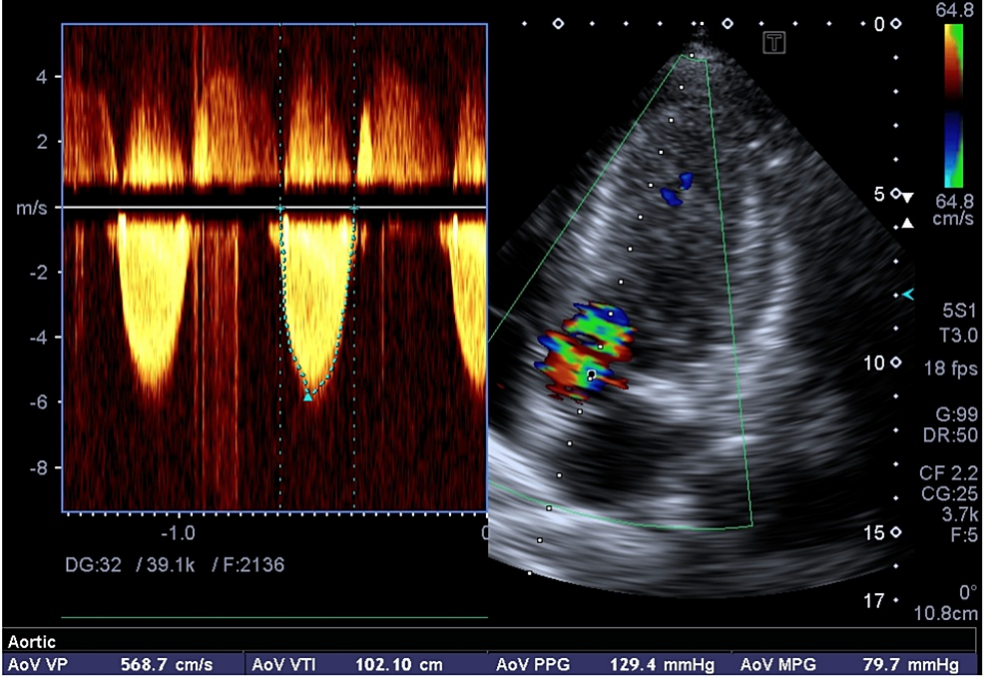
AP5CH view with color Doppler and spectral Doppler image. There is severe LVOT obstruction with peak velocity 5.68m/sec, peak gradient 129mmHg, and mean gradient 79mmHg.There is also spectral Doppler evidence of AR. AP5CH: apical five chamber; LVOT=left ventricular outflow tract; AR=aortic regurgitation
